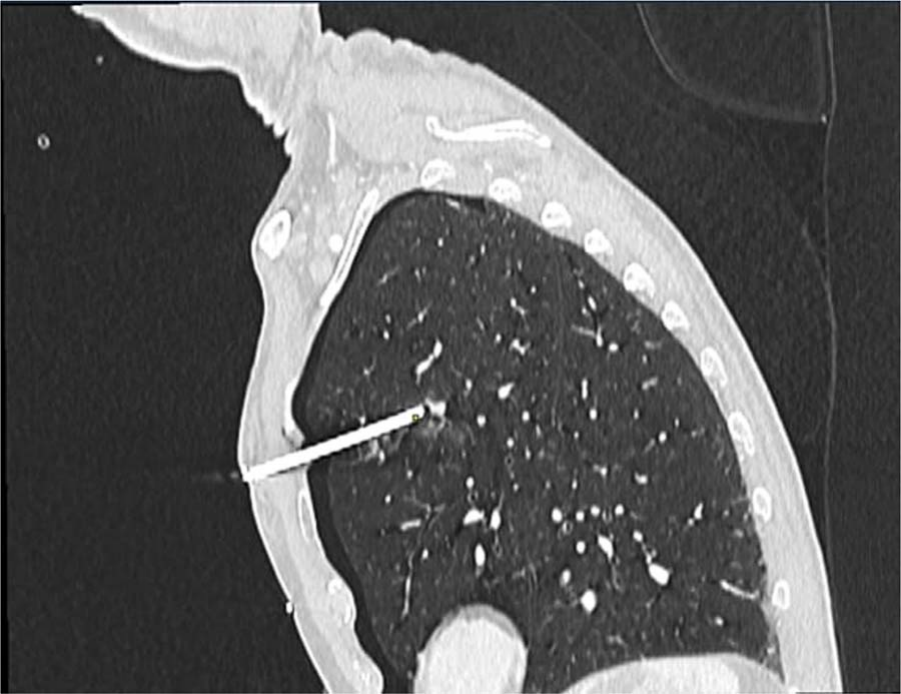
Sagittal CT chest demonstrating nail lodged in the left upper lobe, 3 mm from the anterior segmental branch of the pulmonary artery.
